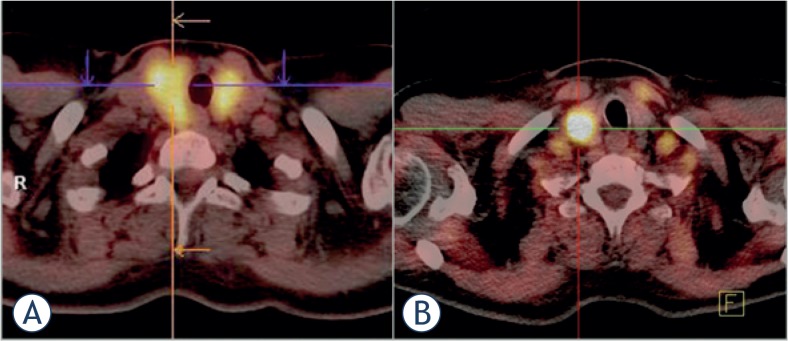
Fusion (PET-CT) scans of the thyroid. A diffuse ^18^F-FDG accumulation in the thyroid is presented on scan (A) (this scan was done at the Institute of Oncology Ljubljana). A focal ^18^F-FDG accumulation in the thyroid is presented on scan (B) (this scan was done at the University Medical Centre Ljubljana).
